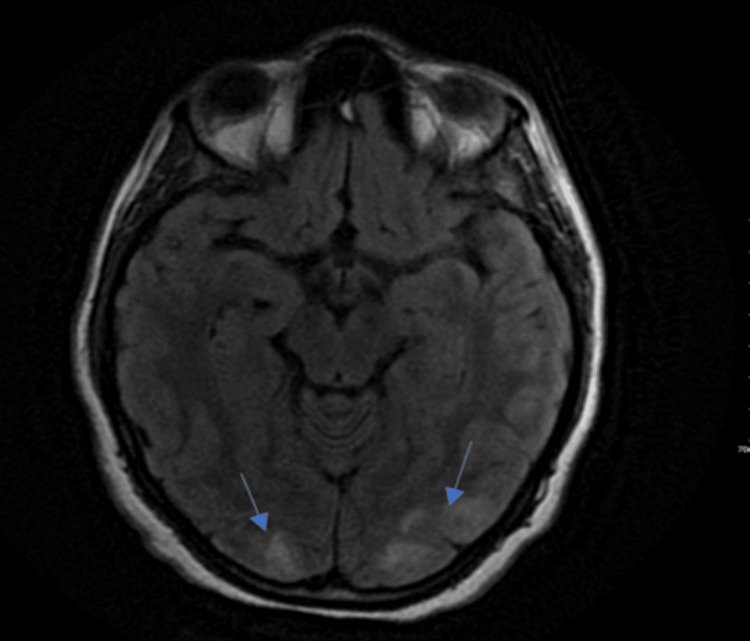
FLAIR MRI showing posterior high signal intensity changes FLAIR: fluid-attenuated inversion recovery
